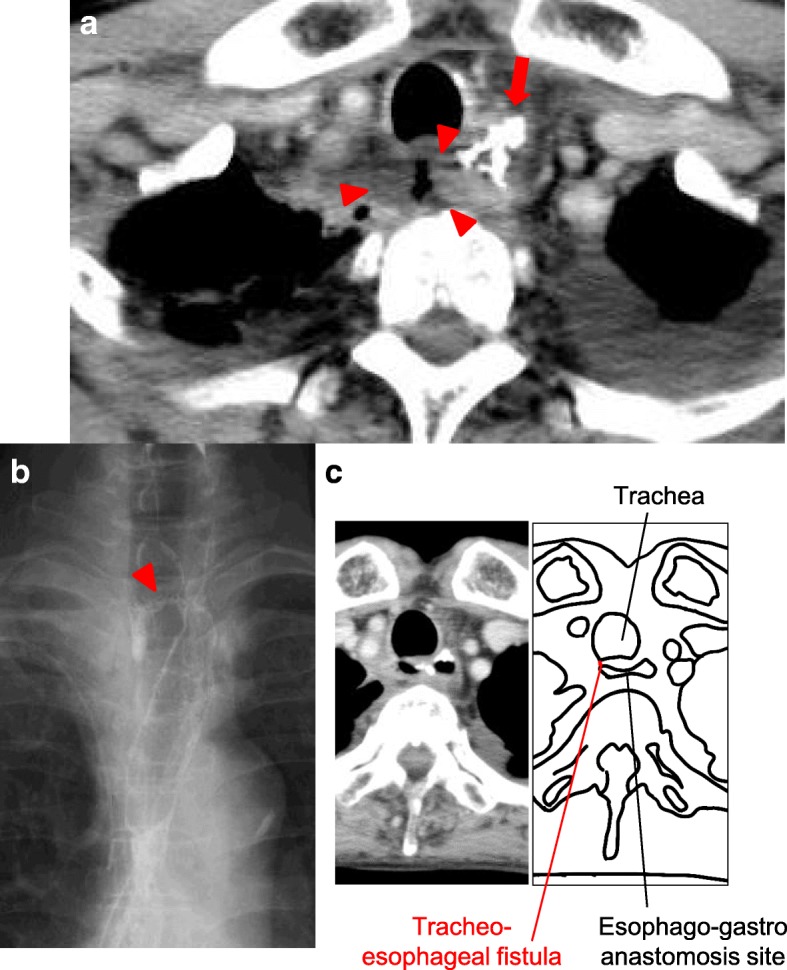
Image findings of postoperative abscess and tracheoesophageal fistula (TEF). a The CT shows the leakage of esophagogastric anastomosis. The arrow indicates the hole of leakage and the arrowheads indicate the abscess. b, c The esophagography shows a TEF. The arrowheads indicate the fistula
